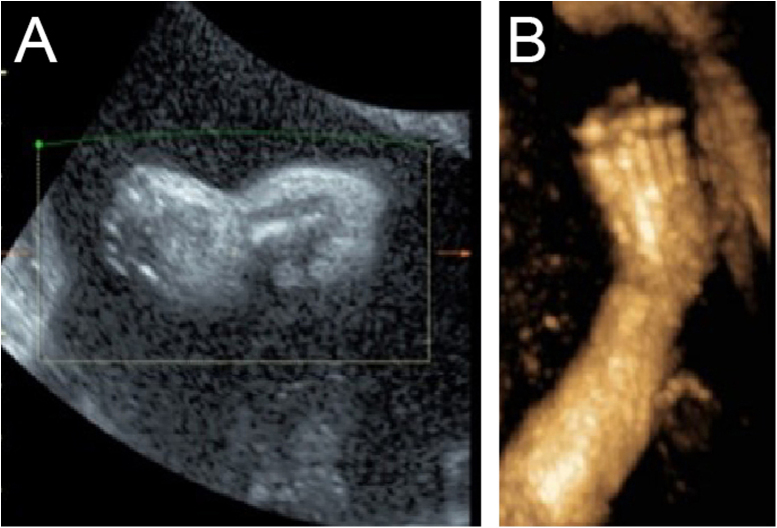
(A) 2-D ultrasound: the image of the right feet of the fetus. (B) 3-D ultrasound: pedal oedema and clubfeet
